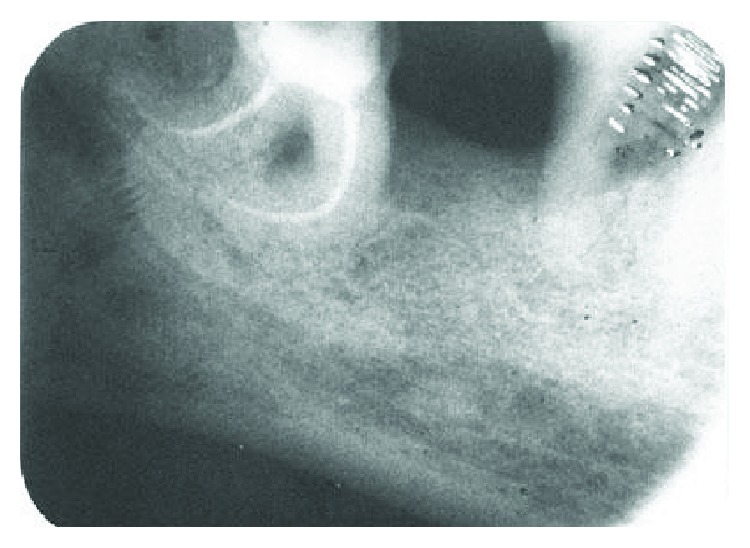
Post obturation radiograph.
